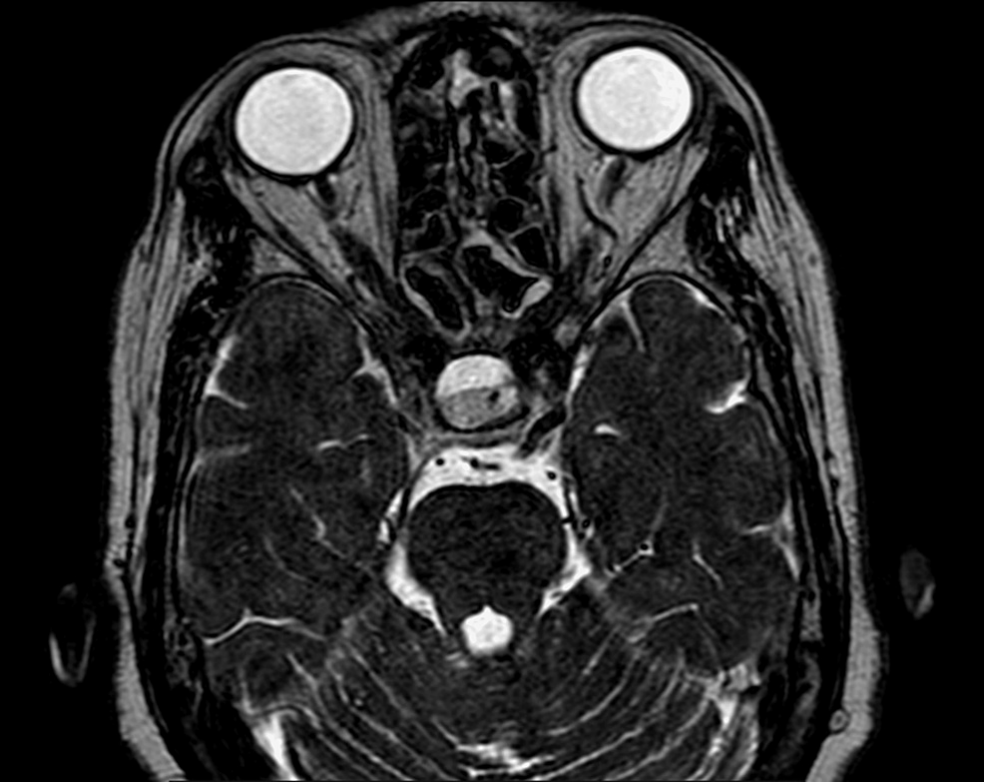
Axial image showing a FLAIR hyperintense lesion in the sellar region
